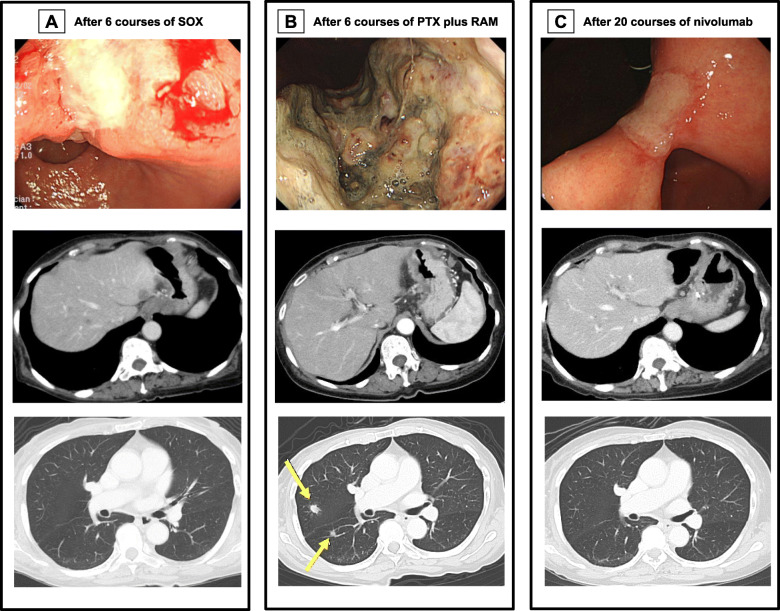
a After 6 courses of S-1 plus oxaliplatin. b After 6 courses of paclitaxel plus ramucirumab. Computed tomography showed lung metastases (arrows). c After 20 courses of nivolumab
